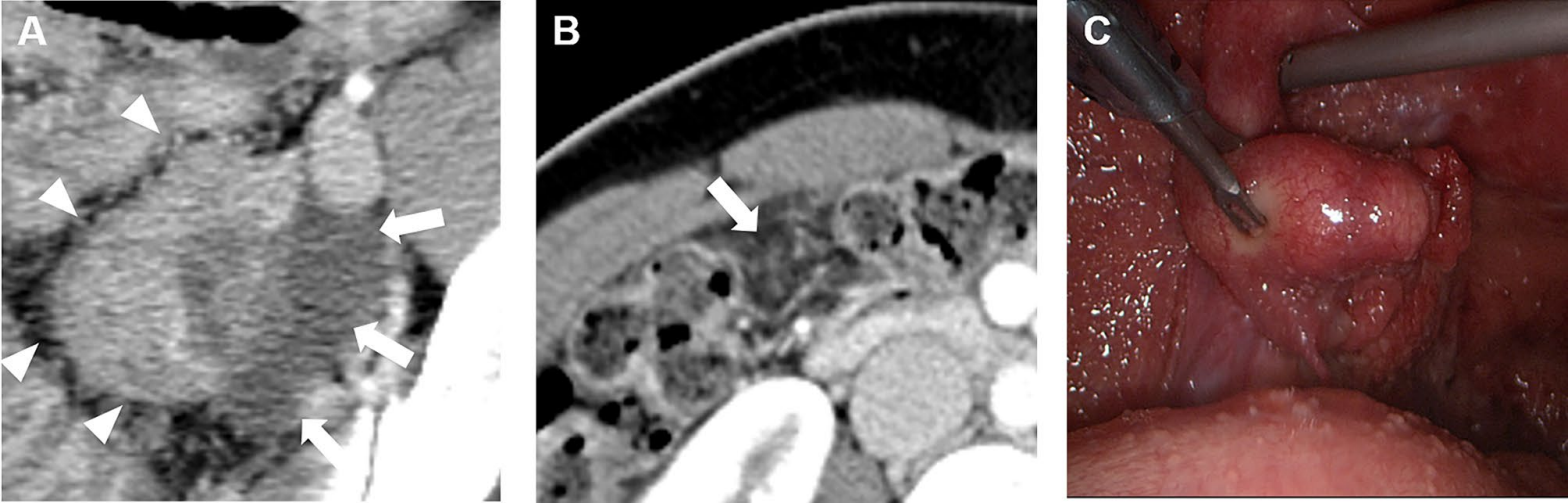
A case of TB peritonitis in a 28-year-old Korean woman. (A) In CT, there were bilateral ovarian masses [Rt. 4.9 cm (not shown), Lt. 4.5 cm (arrowheads)] in the pelvic cavity with hydrosalpinx (arrows). (B) There were diffuse peritoneal infiltration and nodularity without discrete peritoneal nodules or masses > 5 mm, considered peritoneal micronodules (arrow). The levels of CA125 and CA19-9 were 104.4 IU/mL and 1.3 IU/mL, respectively. Laparoscopic bilateral salpingectomy and left ovarian cystectomy were performed. (C) Diagnostic laparoscopy showed multiple miliary nodules on the uterine and peritoneal surfaces.
